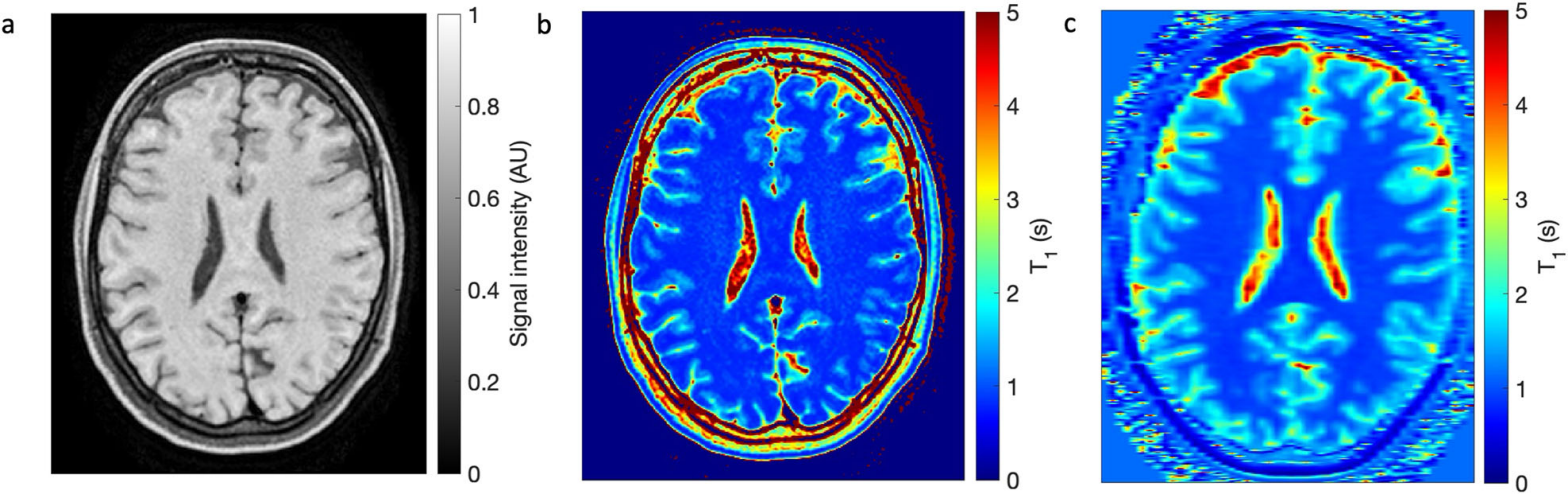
a Three-dimensional T1-weighted TSE image acquired from healthy volunteer #1. b The T1 map derived from the TSE image. c a MP2RAGE T1 map obtained at the same position. MP2RAGE, Magnetization prepared 2 rapid acquisition gradient-echoes; TSE, Turbo spin-echo
